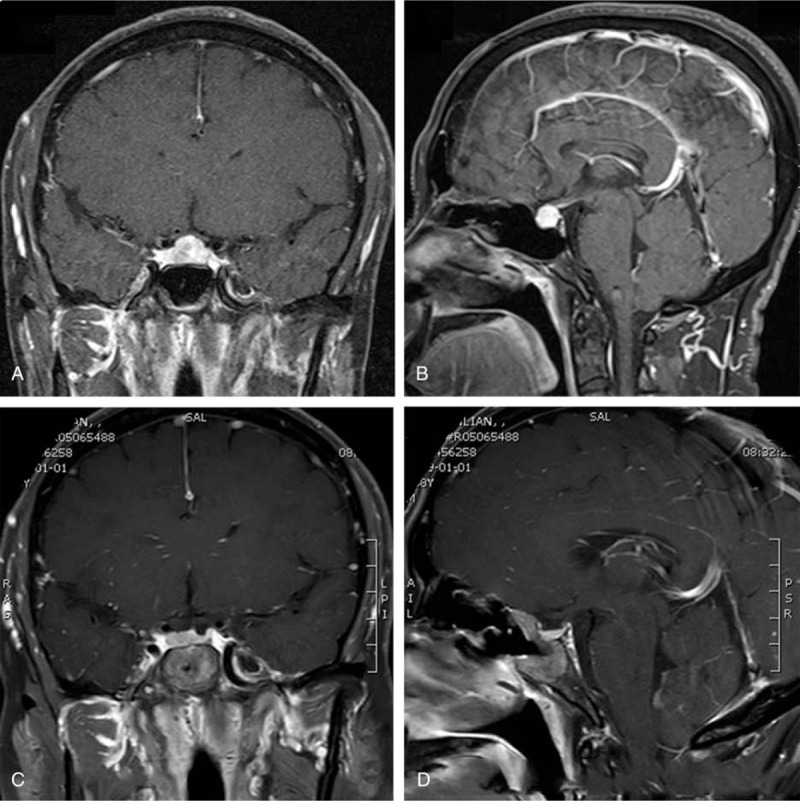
Radiological profiles of Case 1. Intracranial MRI of the patient after treatment (A) coronal and (B) sagittal contrast-enhanced T1-weighted MRI revealed a 1.8 × 1.4 × 1.3 cm sellar mass with remarkable enhancement, and the optic chiasma was oppressed. (C and D) Postoperative MRI showed the mass was partially resected. The optic chiasma is in good shape. MRI = magnetic resonance imaging.
